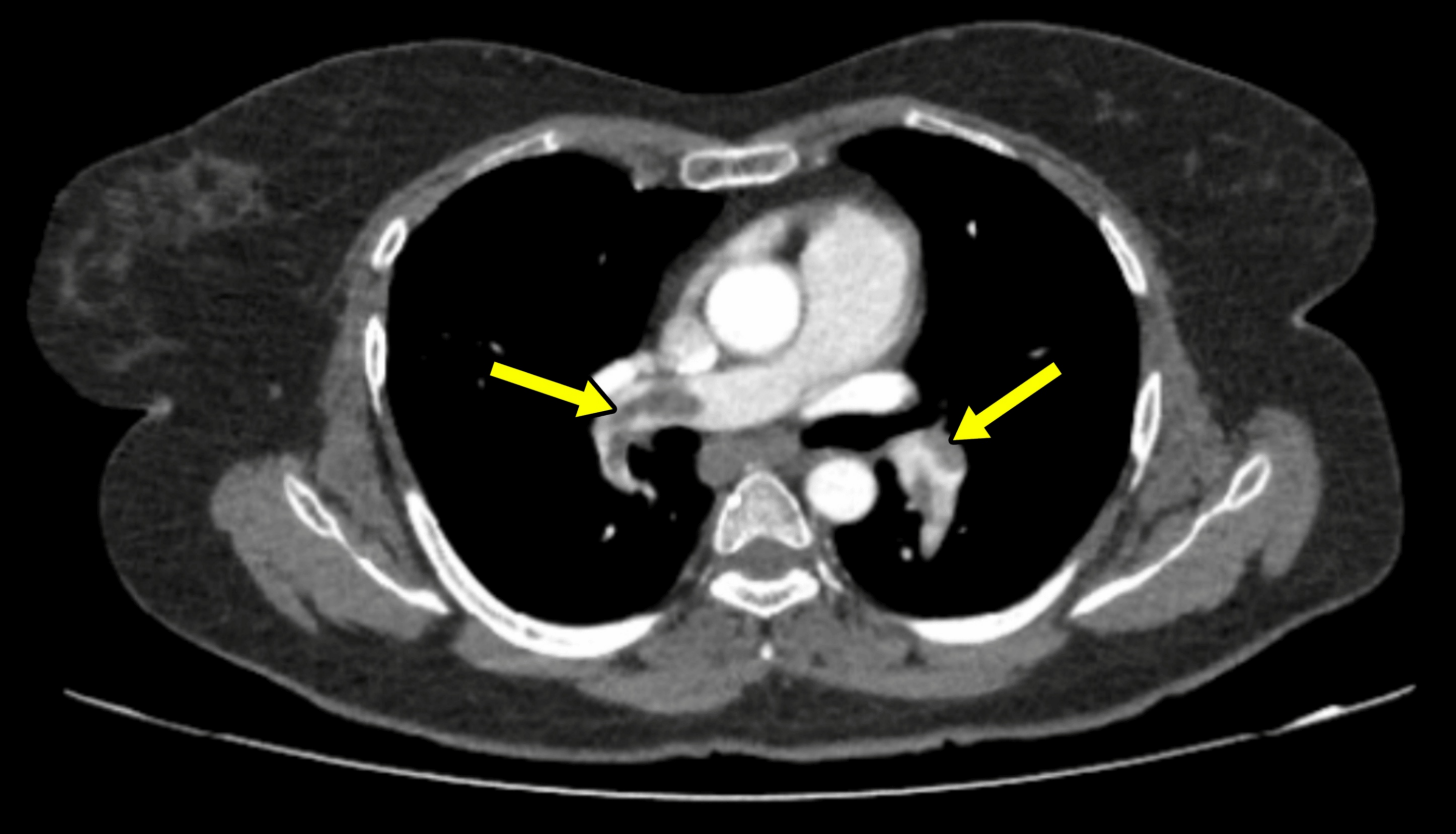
Computed tomography pulmonary angiogram identified filling defects (arrows) in the right and left pulmonary vasculature.
